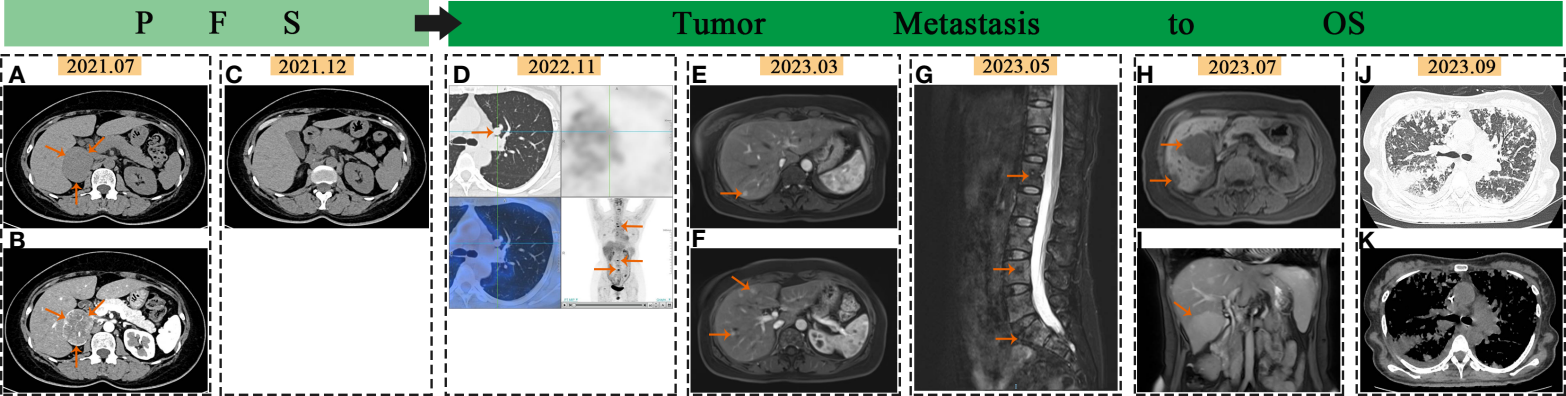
Imaging changes in PCC: In July 2021, the arrows pointed to the adrenal mass on abdominal and enhanced CT scans (A, B). In December 2021, no abnormalities were seen in abdominal CT scans (C). In November 2022, the arrows pointed to lung and bone metastasis on PET-CT (D). In March 2023, the arrows indicated liver metastases on liver MR images (E, F). In May 2023, the arrows pointed to spine metastases on MR images (G). In July 2023, the arrows indicated liver metastases on liver MR images (H, I). In September 2023, CT scan showed extensive lung metastases with inflammation (J, K).
